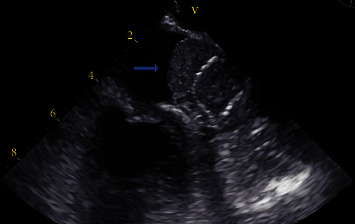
Transesophageal echocardiogram indicating a large thrombus located at the opening of the left atrial appendage covering an implanted WATCHMAN FLX™ device.
